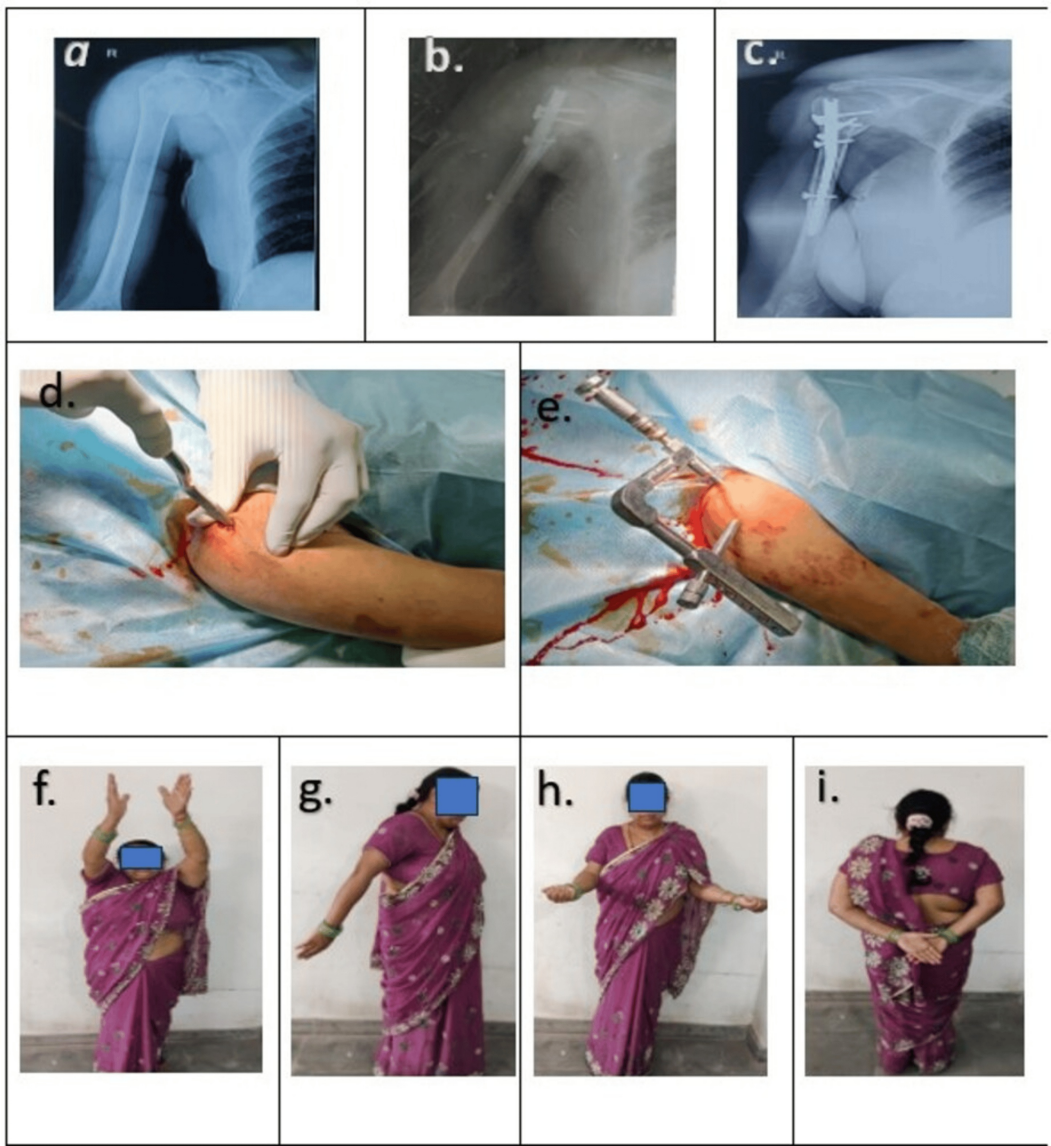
Patient with PHF treated by PHN (a) Preoperative X-ray of the right shoulder joint; (b) postoperative X-ray; (c) six months follow-up X-ray; (d) entry made with bone awl; (e) proximal locking done through jig with the help of a sleeve; (f, g, h, i) functional outcome PHF: Proximal Humerus Fracture; PHN: Proximal Humerus Interlocking Nail
